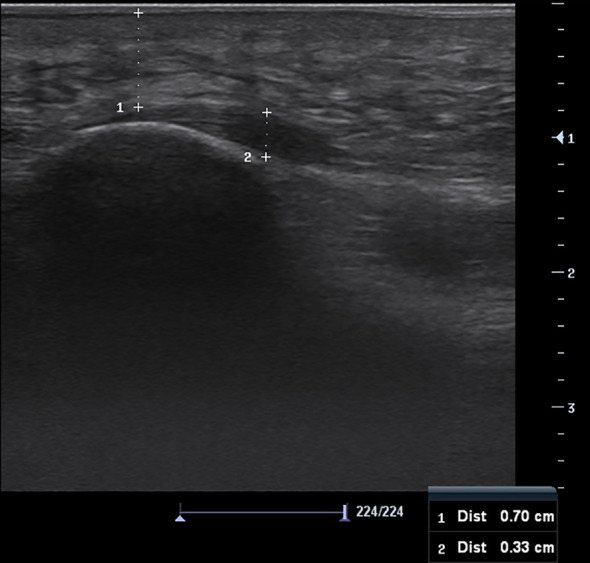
Ultrasound image under loaded conditions. Measurement 1 corresponds to plantar fat pad thickness, while measurement 2 corresponds to plantar fascia thickness at its proximal insertion on the calcaneus.
